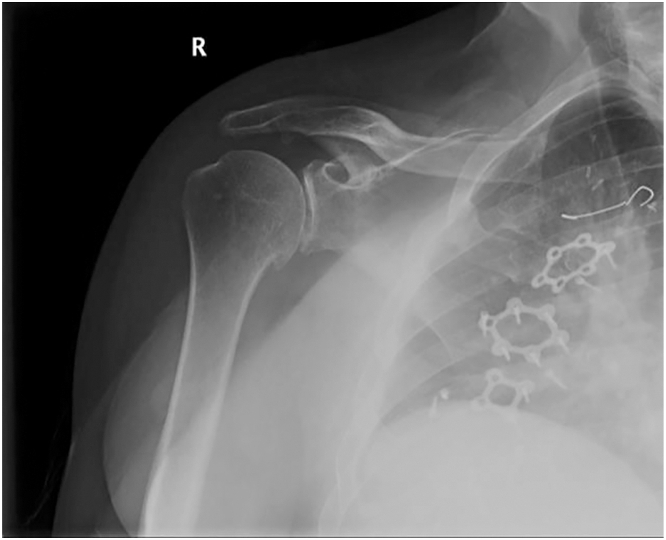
Anteroposterior view of a right shoulder roentgenogram that incidentally detected displaced screws and potentially displaced plates. (R, right.)
